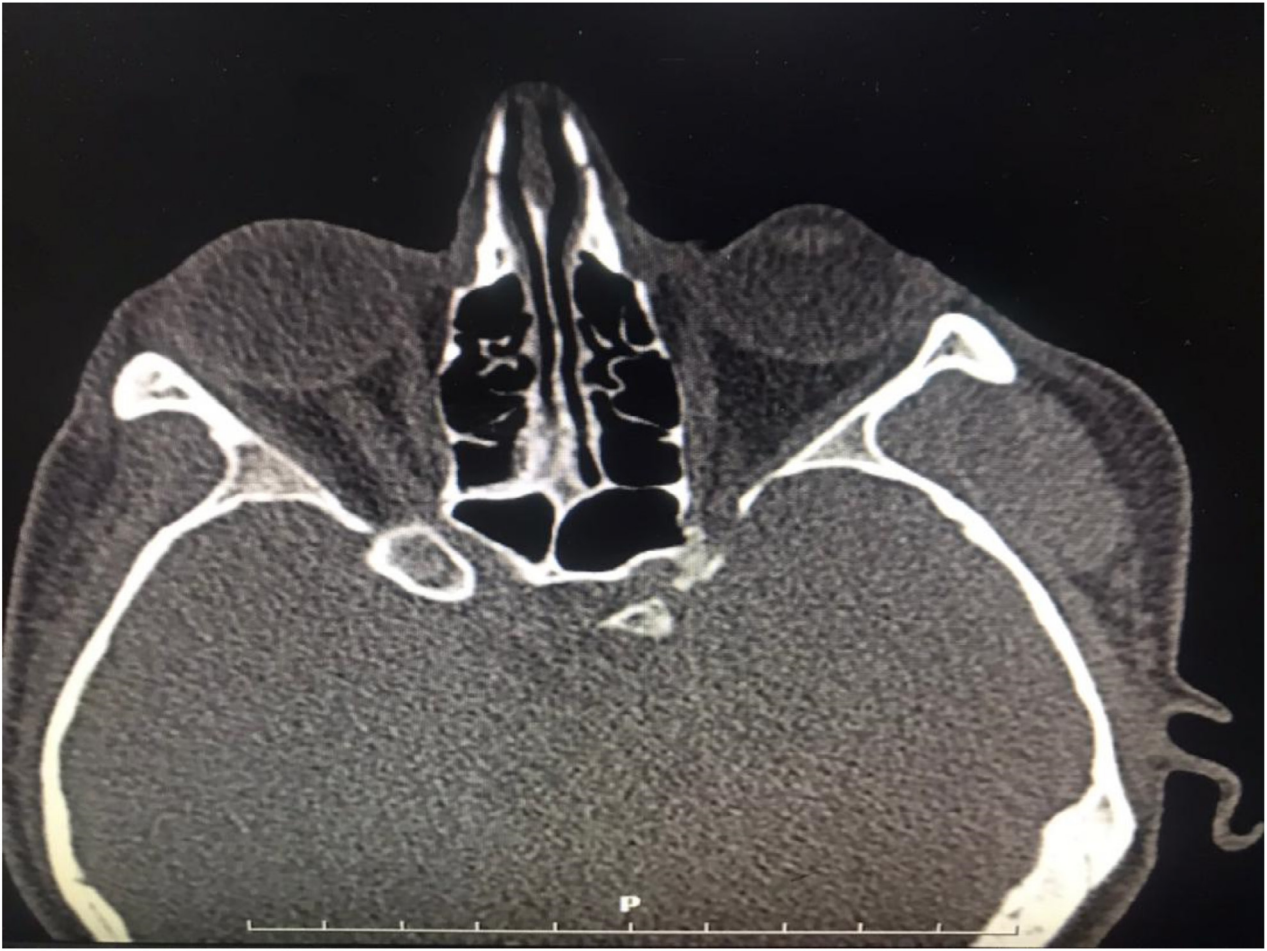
Computed tomography scan showing a mass lesion in the left temporalis muscle.
